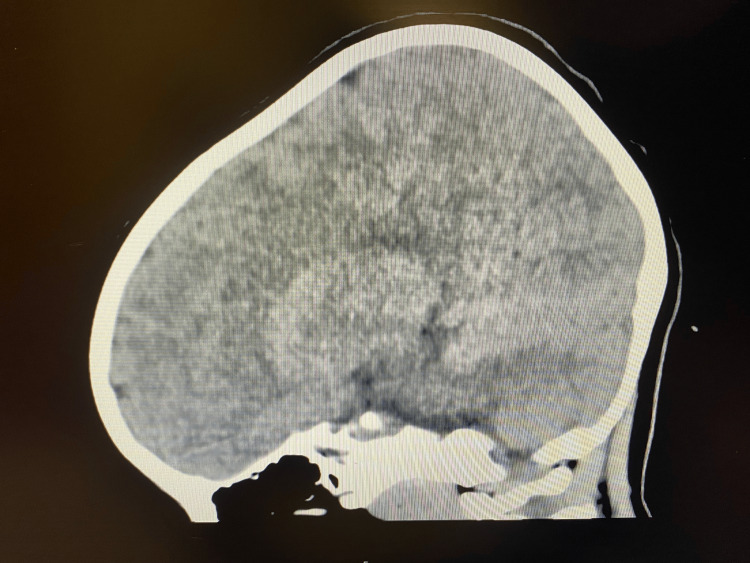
Non-contrasted computed tomography of the head in sagittal view
